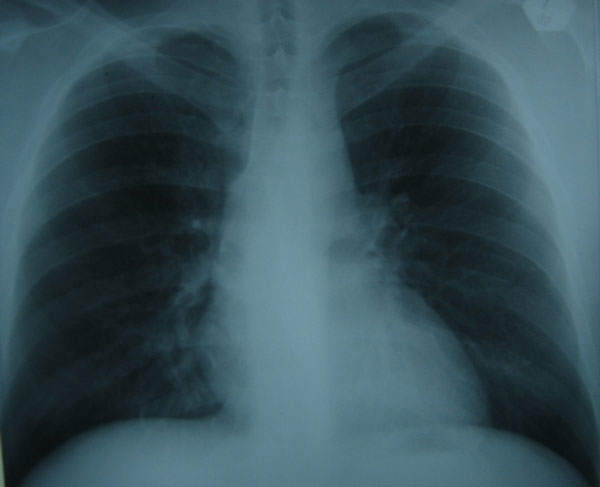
Posterolateral Chest radiography of the patient was essentially normal.
